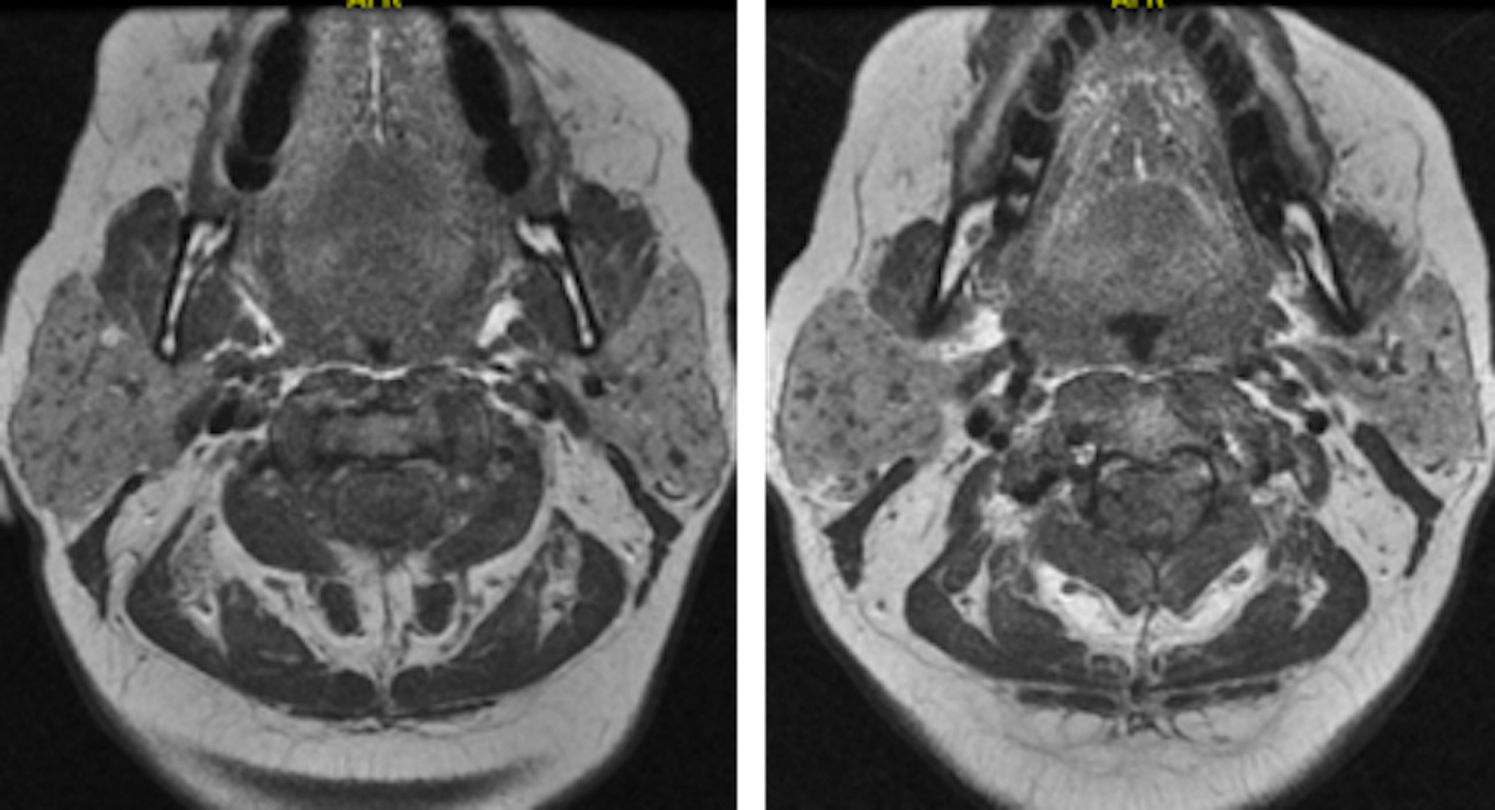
MRI brain with and without contrast. T1-Weighted images in sequential order craniocaudally (right to left image) shows enlarged parotid glands bilaterally, more pronounced on right side with nodular ductal dilations and mixed calcifications. This MRI also shows contrast enhancement of the tympanic segments of bilateral facial nerves, compatible with facial neuritis.
